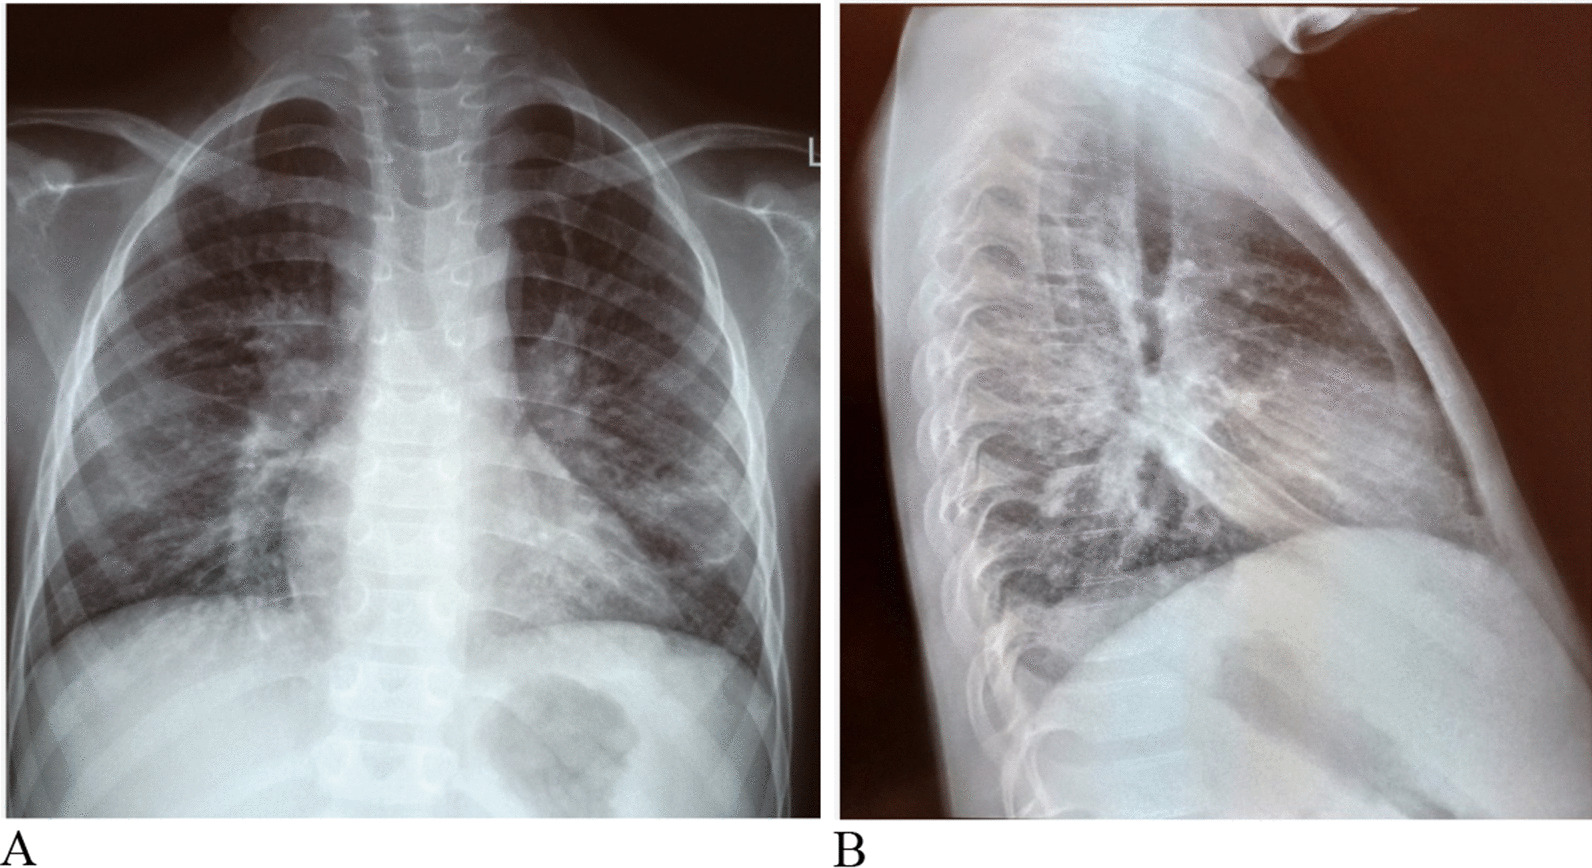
Chest radiograph. A Antero-posterior view, B lateral view, showing normal mediastinal and hilar regions.
